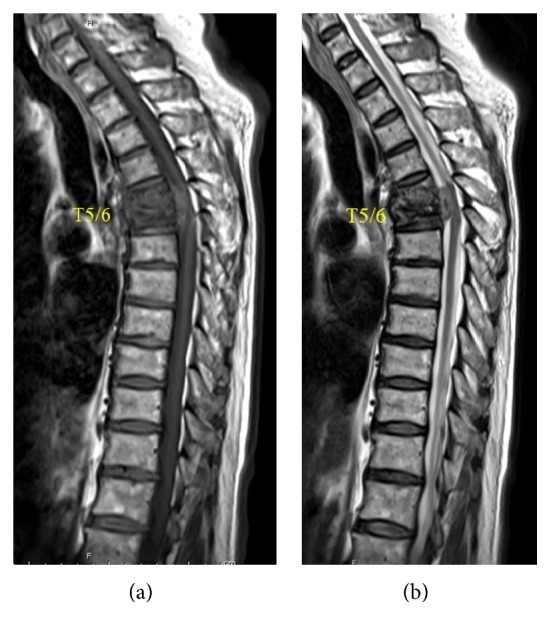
(a) Sagittal T1-weighted and (b) sagittal T2-weighted magnetic resonance imaging of the thoracic spine shows destruction of the T5 and T6 vertebrae and an epidural soft tissue mass with anterior dural sac compression.
